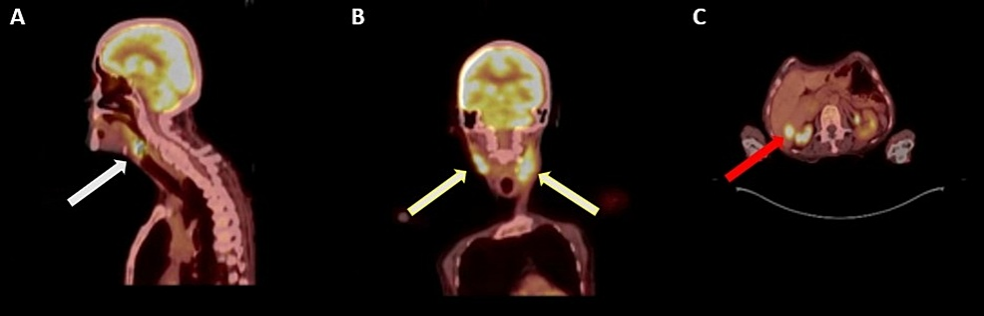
Staging positron emission tomography (PET)/CT (A) It demonstrates primary laryngeal mass (white arrow). (B) There is bilateral hypermetabolic cervical adenopathy (yellow arrow). (C) Section delineates liver metastasis (red arrow).
